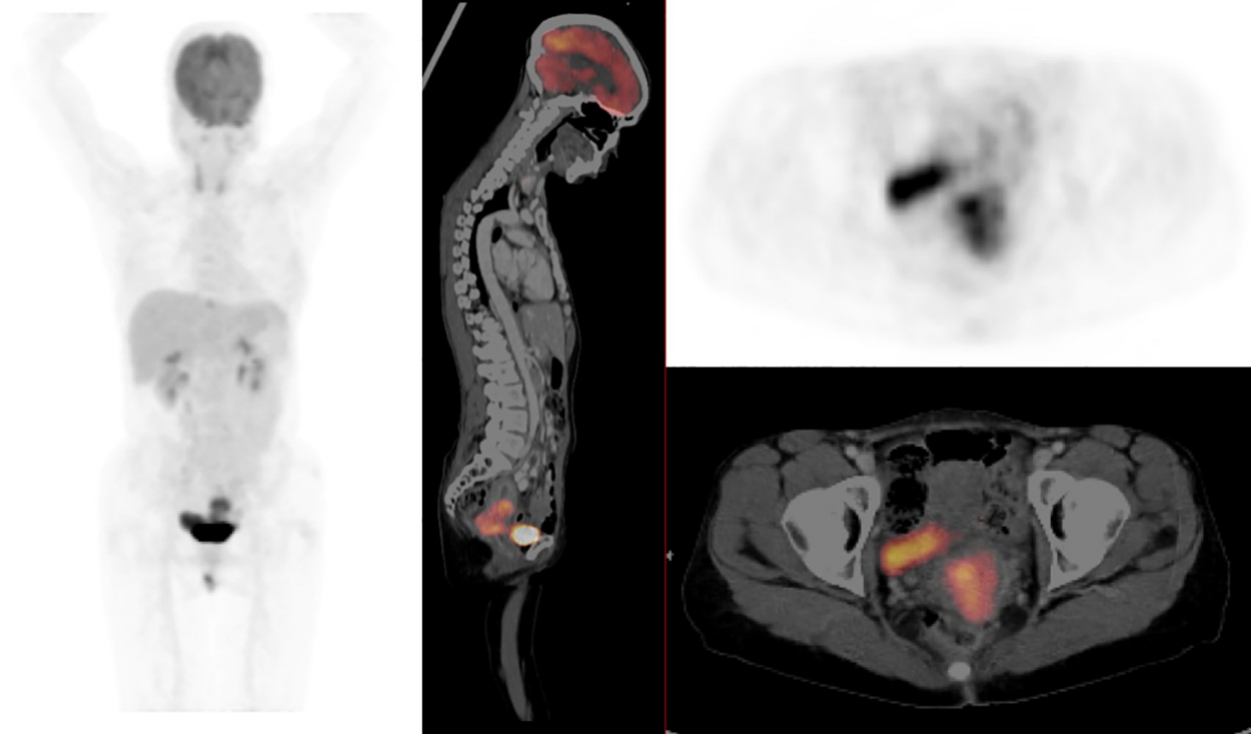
FDG-PET/CT images of a 46-year-old HIV-infected female referred for imaging with stage IIB squamous cell carcinoma of the cervix (SUVmax = 13.06, SUVmean = 4.22, MTV = 186.66, and TLG = 787.77). (A) Coronal PET, (B) sagittal fused PET/CT, (C) axial PET and (D) axial fused PET/CT images show disease localized to the cervix with no distant metastasis.
